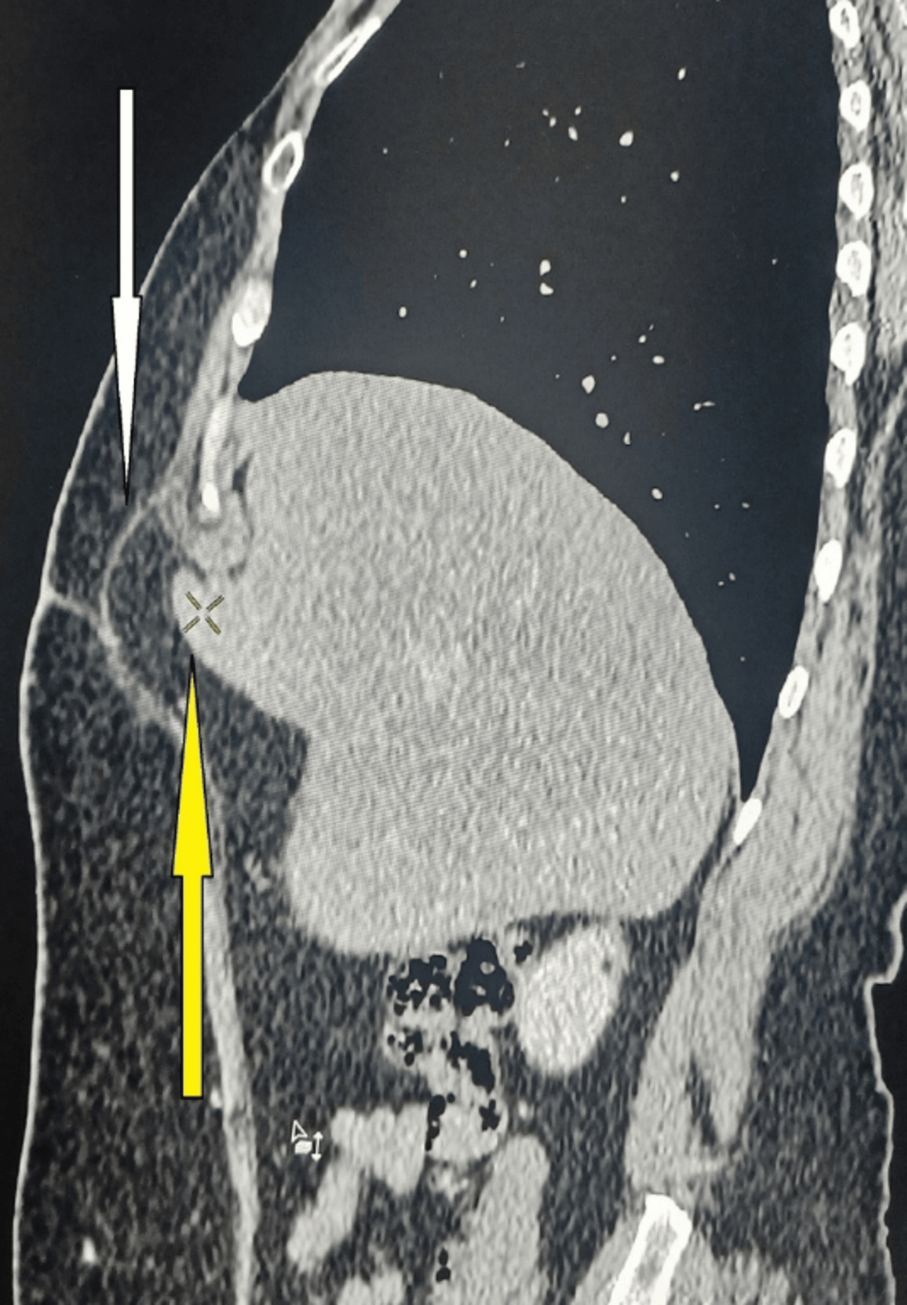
Sagittal section of CT scan abdomen showing hernial sac through surgical scar (white arrow) and herniation of liver into the hernial sac (yellow arrow)
